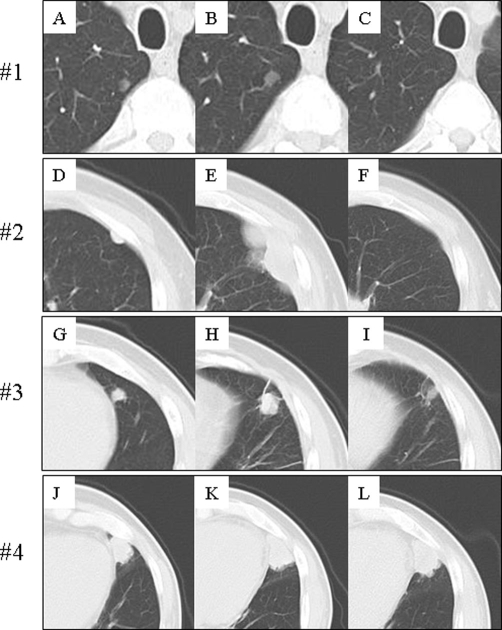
Chest CT before and after the combination therapy with IFN-alpha and capecitabine. Pulmonary metastases were found 8 months after radical nephrectomy (A,D,G,J). Combination therapy with IFN-alpha and UFT failed to achieve tumor responses and PD was observed (B,E,H,K). Two (#1, #2) out of four pulmonary metastatic sites disappeared completely, one site (#3) showed over 50% decrease in size, and the remaining one site (#4) did no change in size after 2 courses of the combination therapy with IFN-alpha and capecitabine (C,F,I,L).
